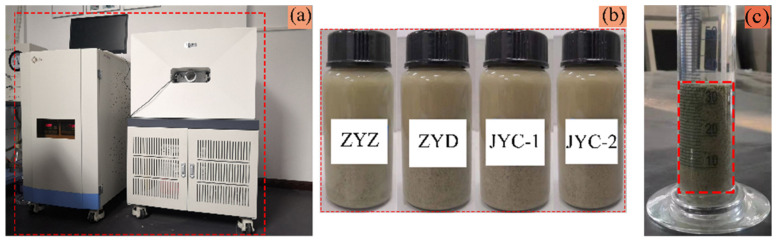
Nuclear magnetic resonance observation experiment: (a) MRI analyzer; (b) samples of floc solution; (c) floc extraction location.
